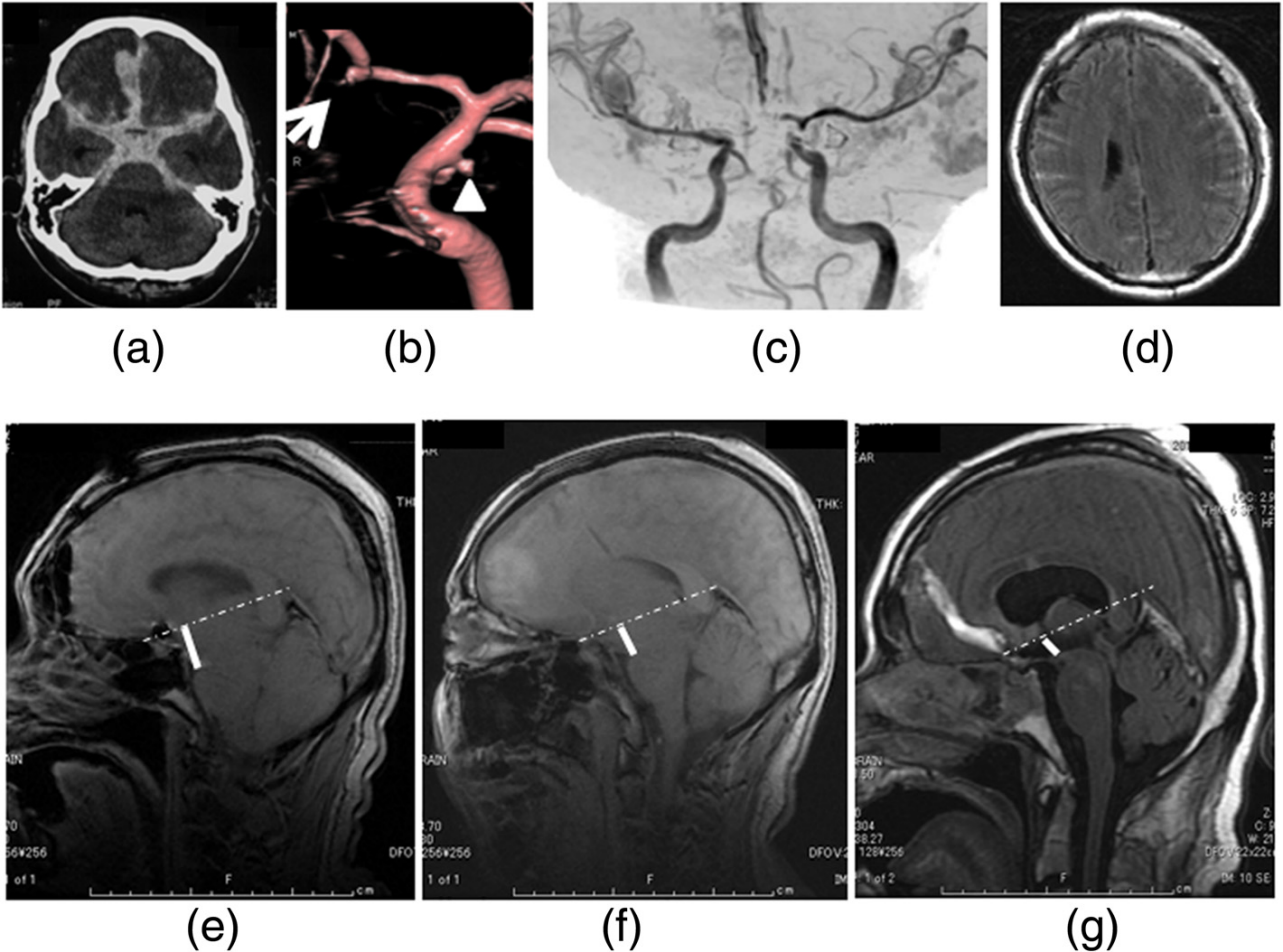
Clinical course of the case. (a) Computed tomography (CT) scan showing diffuse, thick SAH and hematoma in the right frontal lobe. (b) DSA showing a left IC-PC aneurysm (arrowhead) and another suspected aneurysm at A-com (arrow). (c) MRA showing no severe vasospasm. (d) MRI showing fresh subdural hematoma (e) T1 sagittal MRI (day 4) showing marked brain sagging with mild tonsillar descent. A dotted straight line is drawn from the tuberculum sellae to the confluence of the straight sinus. A thick line is drawn indicating the distance from the mammillary body to the line. (f) T1 sagittal MRI (day 15) showing the shorter distance than that of day 4. (g) T1 sagittal MRI (day 21) showing the shorter distance than that of day 15. SAH, subarachnoid hemorrhage; DSA, digital subtraction angiography; IC-PC, internal carotid artery-posterior communicating artery; MRA, magnetic resonance angiography; MRI, magnetic resonance image.
